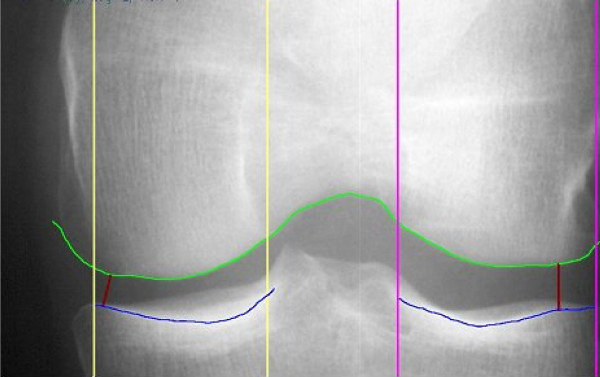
A digitized radiograph analyzed for minimum joint space width using an automated computer algorithm (medial compartment on left).
